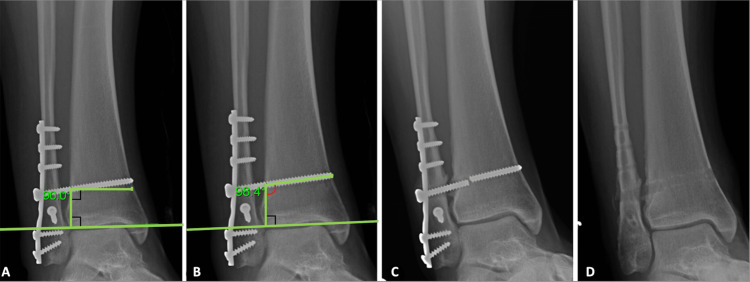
Series of radiographs All radiographs are from a mortise view. The patient was a 20-year-old female with a BMI of 33.7 who suffered a distal fibula fracture with syndesmotic instability. Surgical fixation was done using a plate and screw construct with a single 4.5 mm syndesmotic screw. Radiograph demonstrating a 90-degree angle with tibial plafond joint reference used to measure screw angle (A). The screw was placed at a 98.4-degree angle in this patient (B). After 57 weeks, a single syndesmotic screw broken within the tibia was seen on follow-up imaging (C). Due to persistent and worsening pain, all hardware, including the syndesmotic screw, was removed (D). No further complications were seen following removal.
